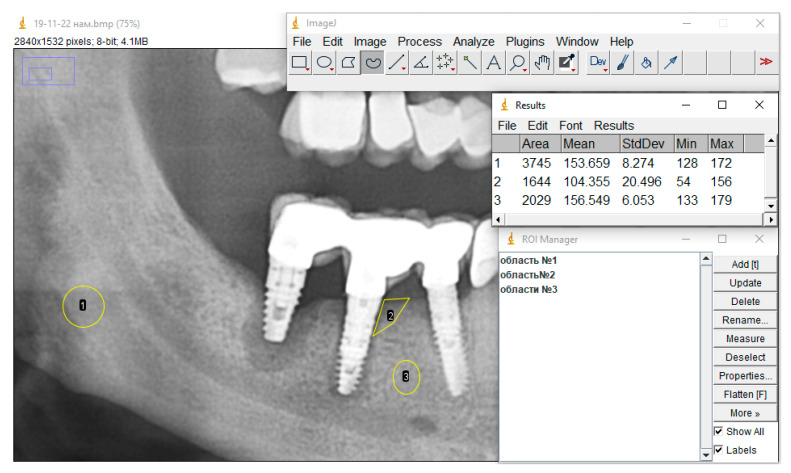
X-ray picture before treatment: area 1—reference, area 2—peri-implantitis, area 3—area without signs of pathological changes in bone tissue.
